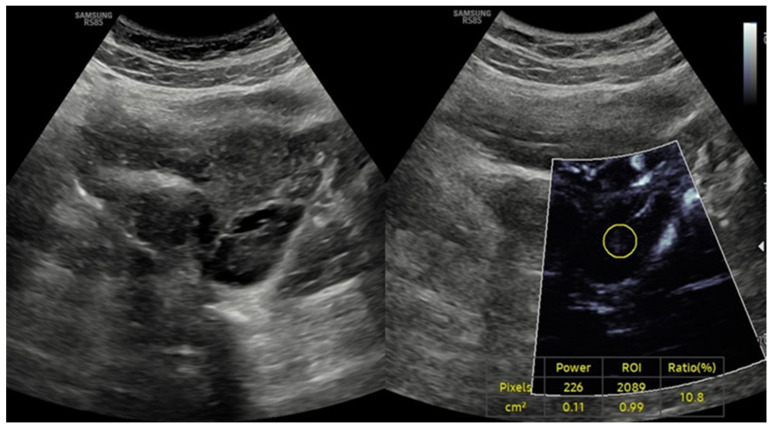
Ultrasound findings in a 26-year-old female patient with isolated polycystic ovarian morphology (PCOM) in the absence of polycystic ovary syndrome (PCOS). Combined gray-scale and MV-Flow Doppler ultrasound images showing multiple small peripheral follicles and a prominent central stroma, consistent with PCOM. The vascularity index (VI), measured on MV-Flow Doppler, is low (ratio: 10.8%), indicating reduced stromal blood flow. The absence of clinical and biochemical hyperandrogenism in this patient supports a diagnosis of isolated PCOM without meeting full diagnostic criteria for PCOS.
